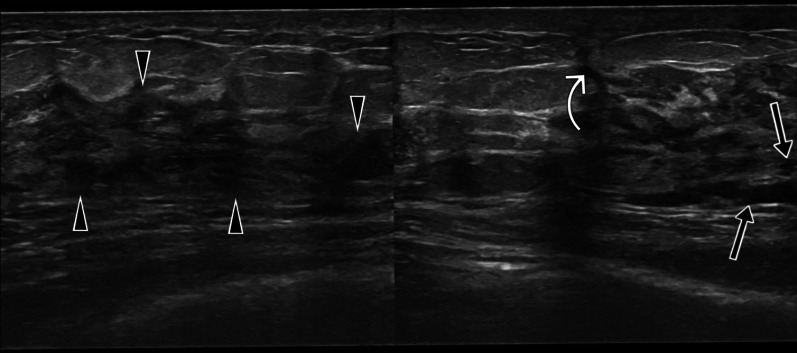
Ultrasound images of a 33-year-old female. Parenchymal diffuse hypoechoic coarsening in the left breast (arrowheads), small fluid collections (arrows), and sinus tract (curved arrow). BIRADS 4
